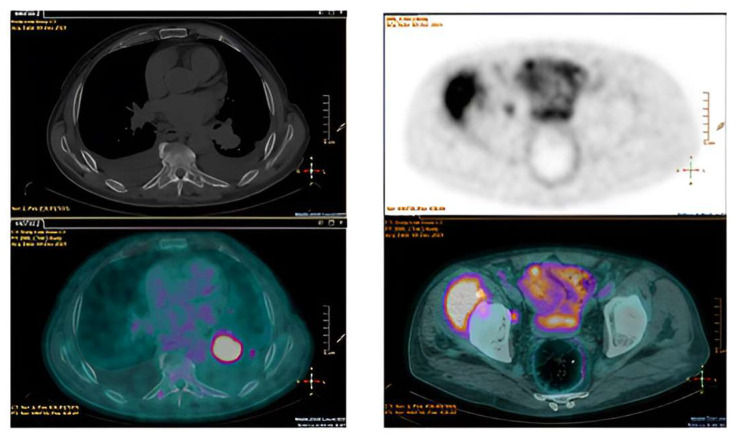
^18^F–FDG–PET/CT scan revealing a significantly abnormal metabolic uptake within a left pulmonary lesion, on the left, also involving adrenal glands, lymph nodes and bone sites, on the right.
